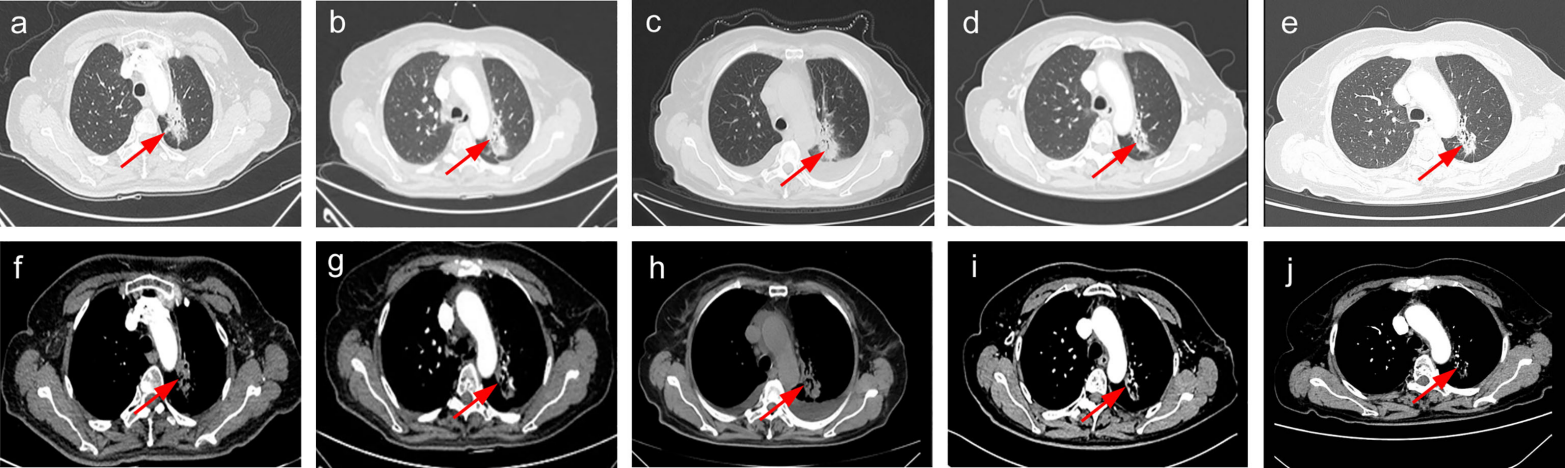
The clinical course according to check CT scan findings. (A, F) Baseline CT scan at diagnosis in April 2020. (B, G) SD on osimertinib in July 2020. (C, H) SD on osimertinib in October 2020. (D, I) PR on aumolertinib in March 2021. (E, J) SD on aumolertinib in July 2021. PR, partial response. SD, stable disease.
